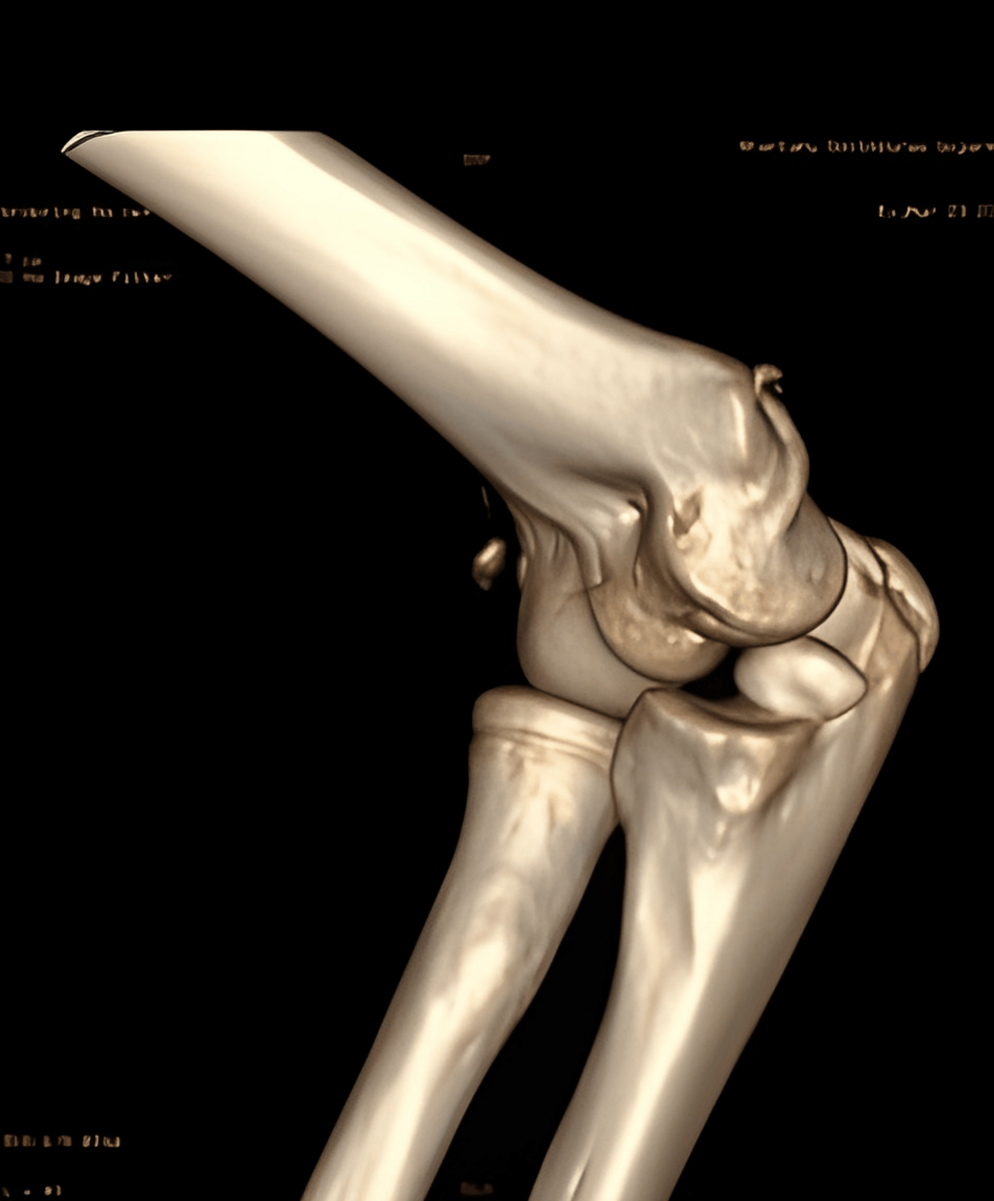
Preoperative CT scan of case 4 showing incarcerated fragment of the medial epicondyle
